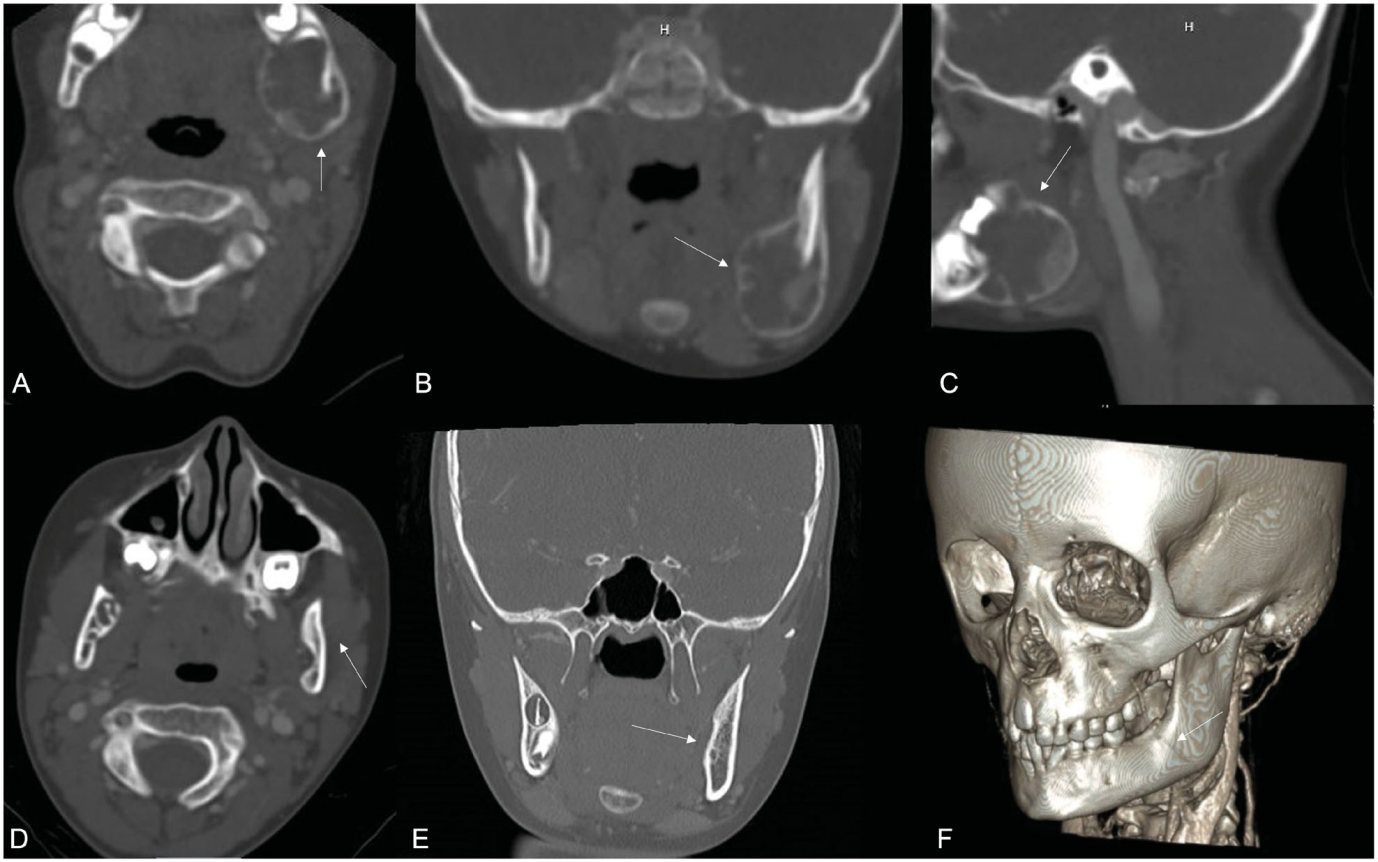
Top row: preoperative CT neck images demonstrating 2 × 2 × 3 cm expansile lesion of the left posterior mandible, mixed radiolucent-radiopaque with trabeculations. (A) axial view, (B) coronal view, (C) sagittal view. Bottom row: postoperative face CT performed three years after surgery. Image demonstrates preservation of the left mandible cortices with normal contours in axial (D), coronal (E), and lateral (3D image for better representation, F) showing no evidence of recurrence.
